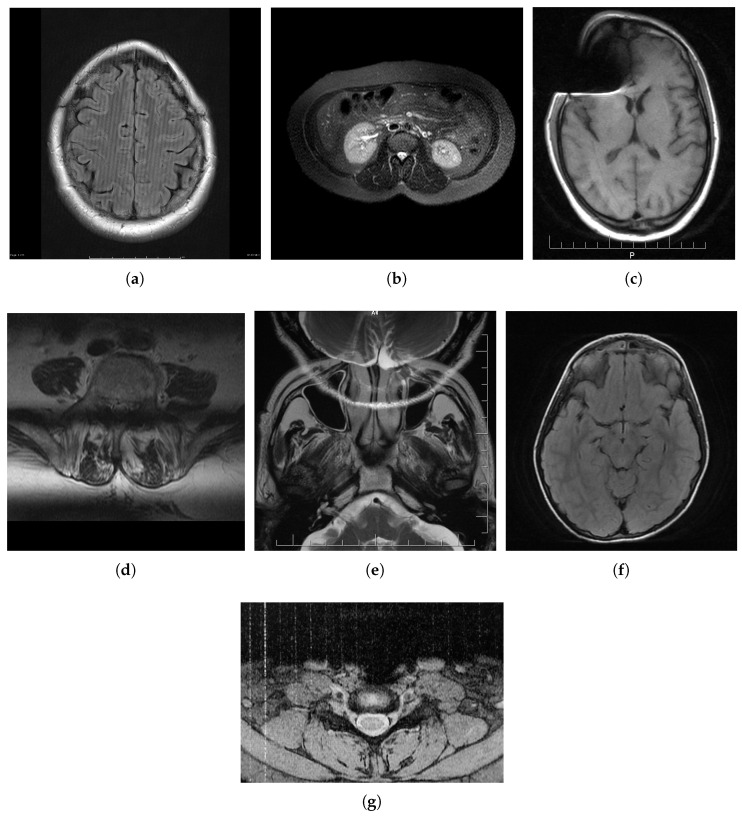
Examples of artifacts in MR images: (a) herringbone; (b) ghosting; (c) magnetic susceptibility; (d) slice overlap; (e) aliasing; (f) Gibbs effect; and (g) zipper. Cases were the courtesy of: (a) Assoc. Prof. Frank Gaillard, rID: 19695; (b) Assis. Prof. Faeze Salahshour, rID: 81727; (c) Dr. Ayush Goel, rID: 22731; (d) Dr. Roberto Schubert, rID: 16705; (e) Dr. Prashant Mudgal, rID: 26927; (f) Dr. Prashant Mudgal, rID: 27302; and (g) Dr. Alan Nazerian, rID: 45665; radiopaedia.org (accessed on 27 April 2022).
